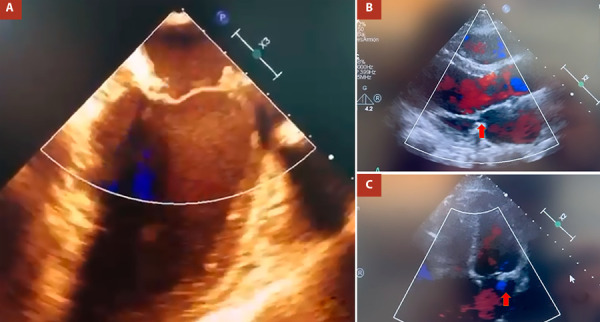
A. Postoperative transesophageal echocardiography showing no mitral valve regurgitation (180o view). B, C. Postoperative transthoracic echocardiography showing minimal mitral valve regurgitation in parasternal and 4 camera views, respectively (red arrow).
